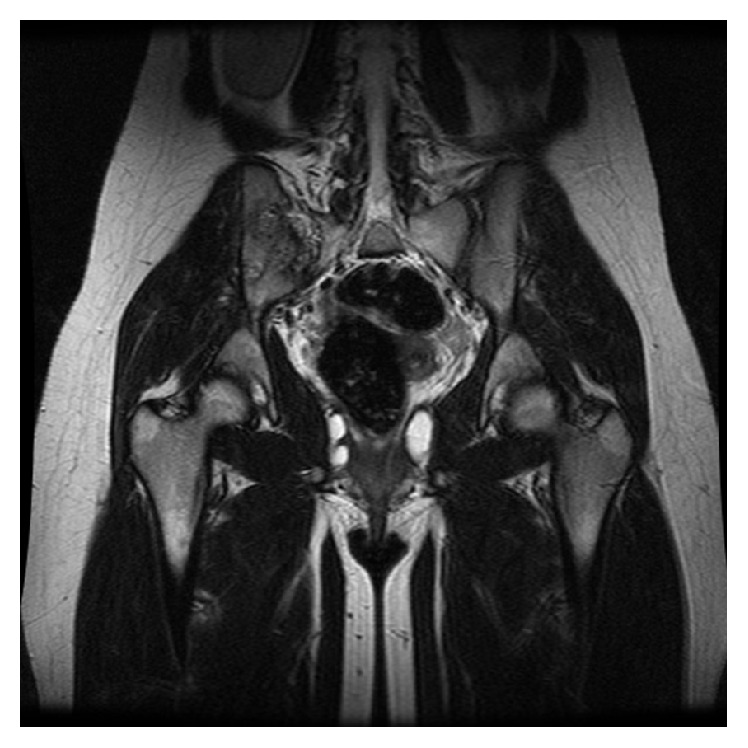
T1-sequence magnetic resonance imaging shows a heterogeneous, lobulated lytic lesion of the right ilium involving the sacroiliac joint.
